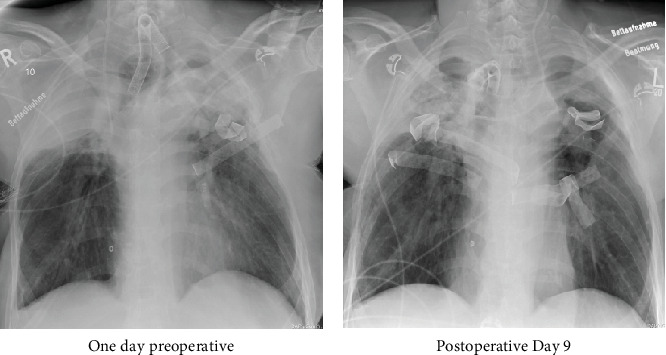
Pre- and postoperative chest x-ray. Legend 4: Chest x-ray on admission with (a) the spherical tracheal balloon of the tracheostomy cannula and (b) the tracheostomy cannula without any enlarged cuff and bilateral wound gauze in the apical thoracic spaces.
